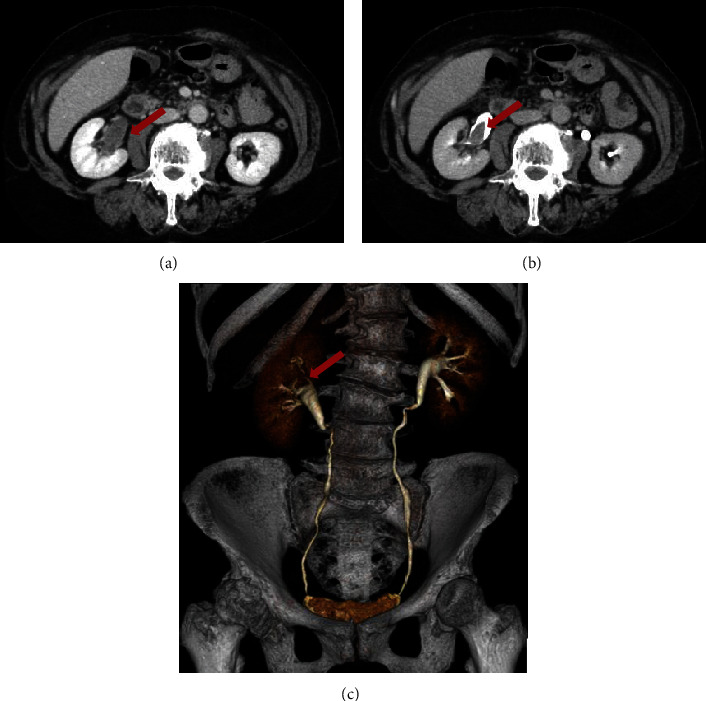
Contrast-enhanced CT reveals an approximately 2 cm mass in the right renal pelvis without evident blood flow. (a) Transverse section of CT delay phase. (b) Transverse section of CT urography. (c) Three-dimensional image of CT urography. CT, computed tomography.
